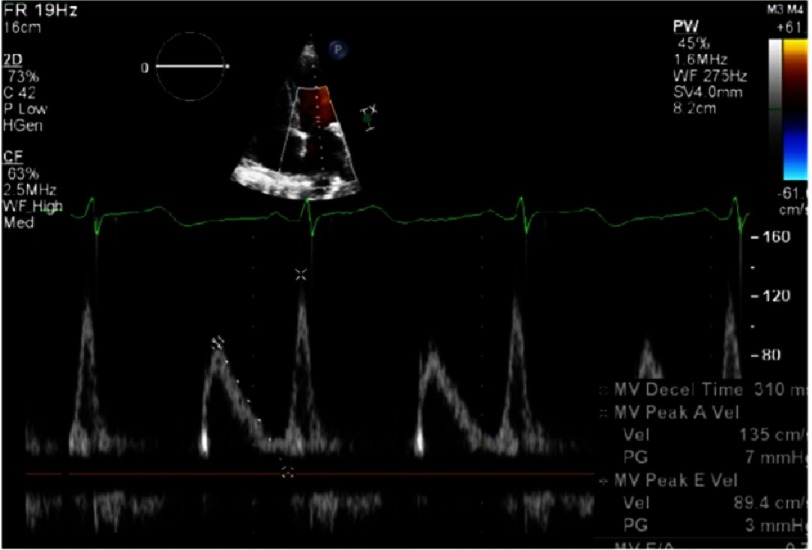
Diastolic dysfunction in early stages, shown by E/A relation <1 in Doppler wave mitral inflow velocities.
